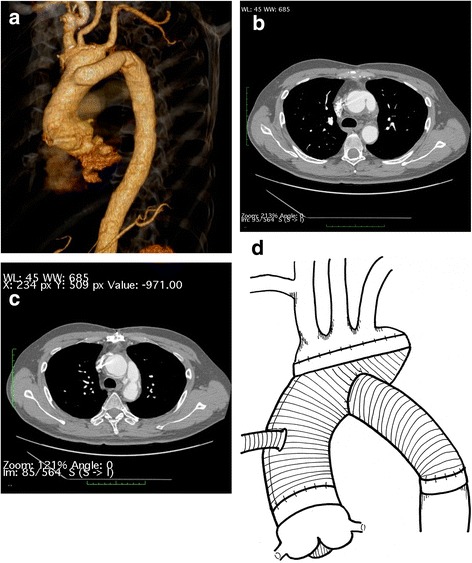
Postoperative multidetector computed tomography (MDCT) showing total aortic arch replacement with island reconstruction using the T-graft technique. It also shows that no false lumens remained after the first surgery. a Synthetic image, (b-c) axial view, and (d) scheme of the procedure. The diameter of the graft: proximal, 30 mm; distal, 18 mm
